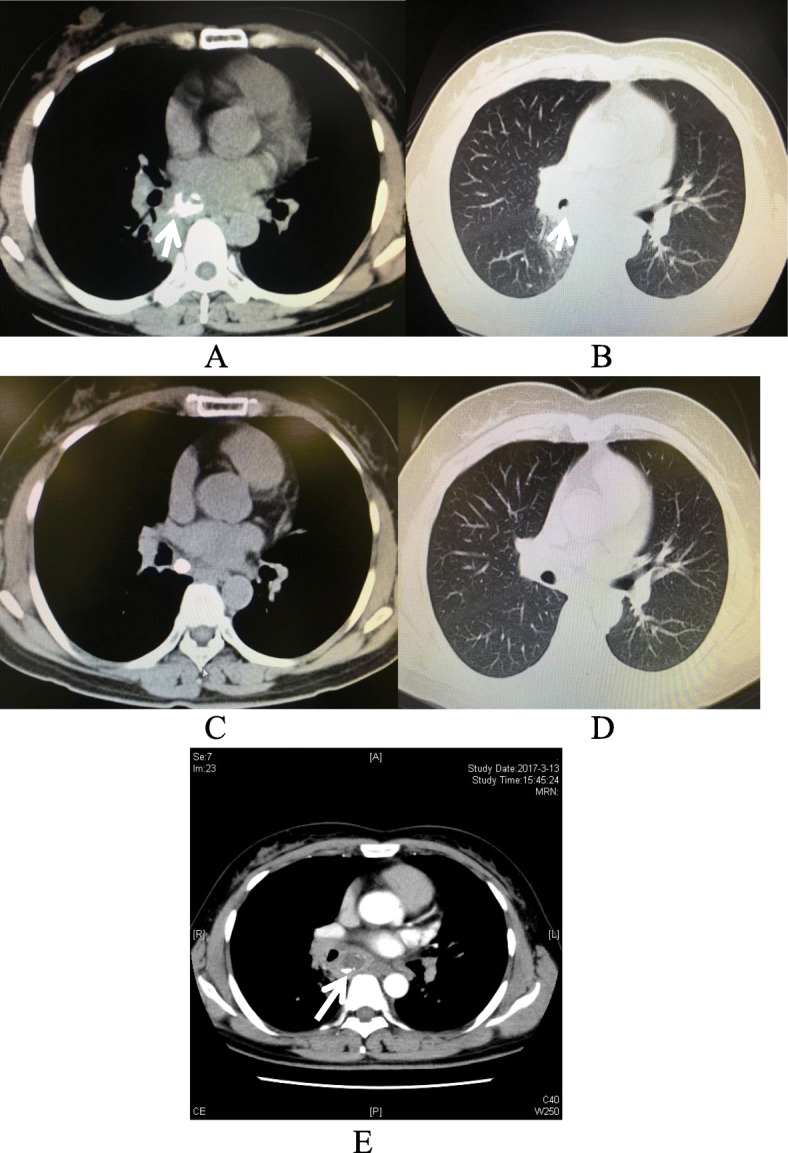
a Computed tomography of the mediastinum showing significantly enhanced signals for mediastinal abscesses with a giant calcified mediastinal lymph node (21 × 18 mm) (arrow). b Chest computed tomography showing a proximal right main bronchial neoplasm (5*4 mm) (arrow) and pneumonia in the right lower lobe. c Computed tomography of the mediastinum 22 months post-discharge showing significantly absorbed mediastinal abscesses with a slimmer calcified mediastinal lymph node (12 × 9 mm). d Chest computed tomography 22 months post-discharge showing complete resolution of pneumonia. e Chest Enhanceed Computed tomography of the mediastinum showing significantly low density for mediastinal abscesses with calcification (arrow)
